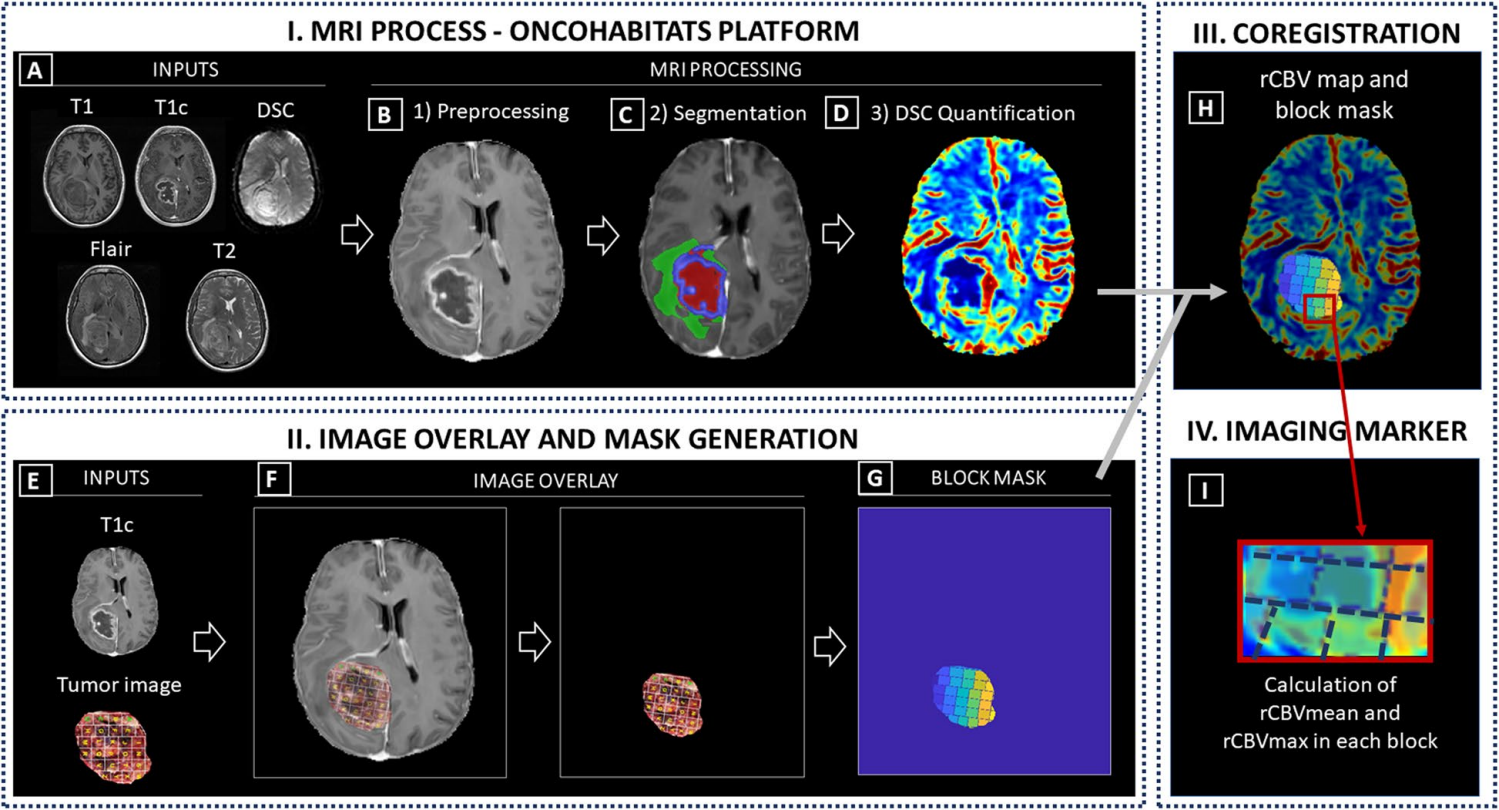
Phases of methodology: I) MRI Process conducted by the Segmentation Service included in the ONCOhabitats platform; II) Image overlay and mask generation; III) Coregistration and IV) Calculation of imaging markers. A Required inputs for the MRI process (T1, T1c, T2, Flair and DSC); (B) Preprocessing of the MRIs; (C) Classic segmentation of the lesion into tumor, edema and necrosis; (D) DSC perfusion quantification and rCBV map calculation. E Inputs to generate the block mask; (F) Image overlay process for mask generation; (G) Block mask; (H) Overlay of the rCBV map and block mask; (I) Calculation of imaging markers (rCBV_mean_ and rCBV_max_) in each tissue block
